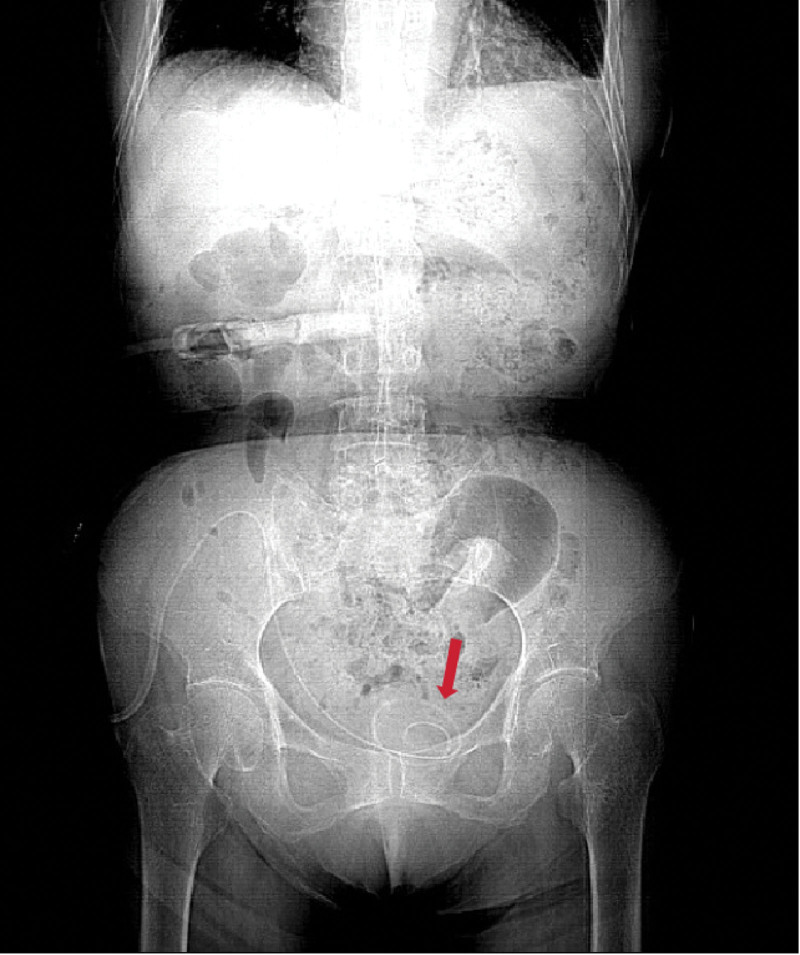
The X-ray indicates that the catheter is in the correct position.
